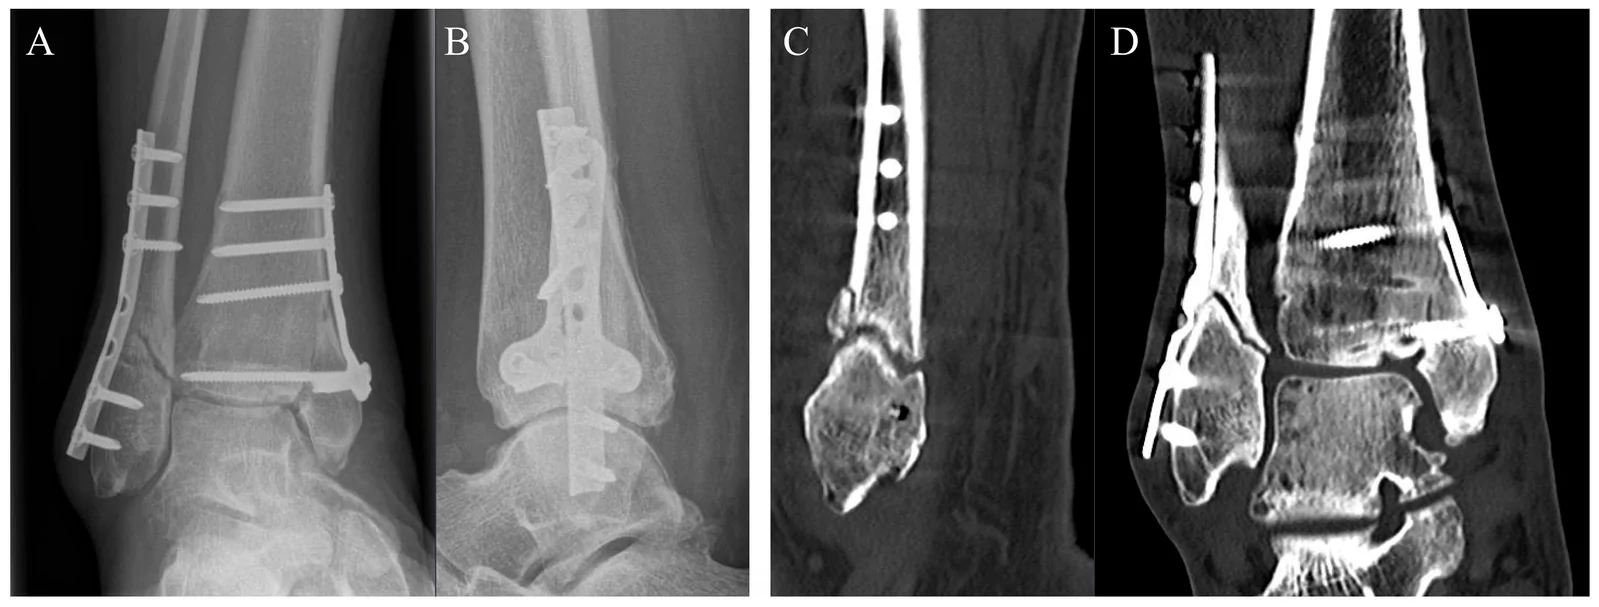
Imaging of the nonunion patient performed 6 months after surgery. Radiographs in (A) AP and (B) lateral views. CT scans shown in (C) sagittal and (D) coronal views.
